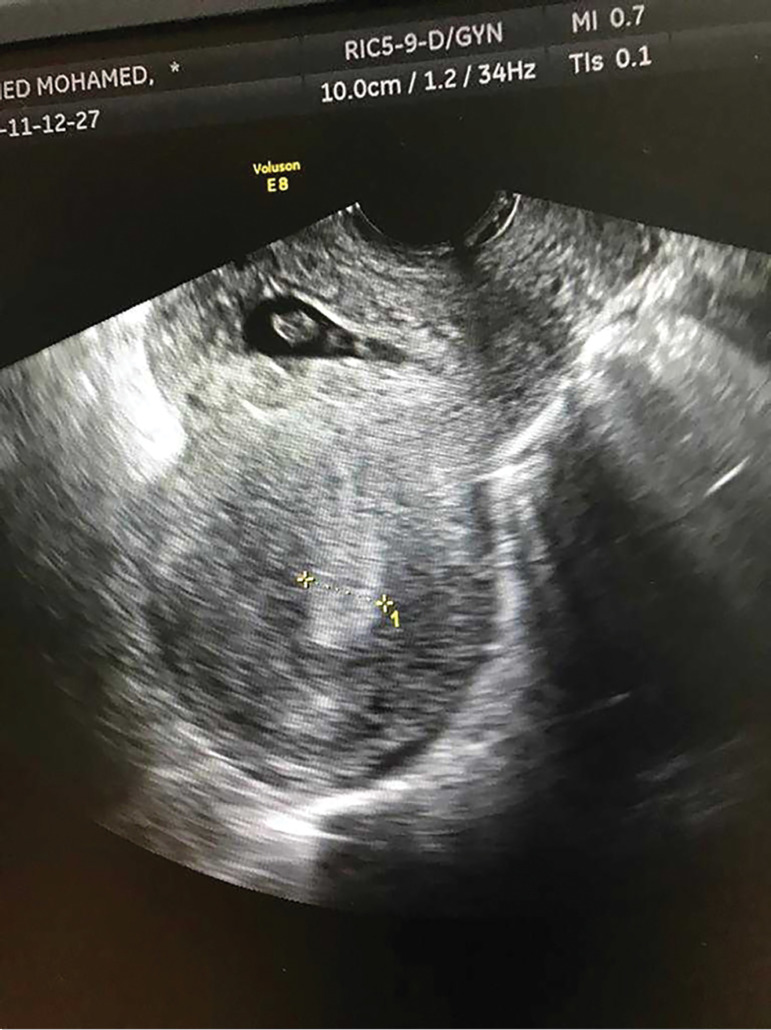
Ultrasound picture showing ballooning of cesarean scar by a pregnancy sac
